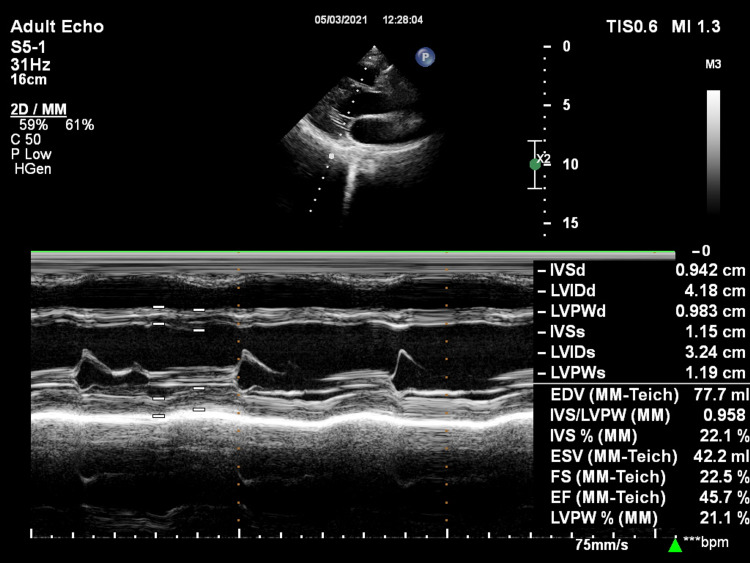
Motion-mode image in the parasternal long-axis view showing estimation of left ventricle ejection fraction. IVSd: interventricular septum diastole; LVIDd: left ventricular internal dimension diastole; LVPWd: left ventricular posterior wall diastole; IVSs: interventricular septum systole; LVIDs: left ventricular internal dimension systole; LVPWs: left ventricular posterior wall systole; FS: fractional shortening; EF: ejection fraction
